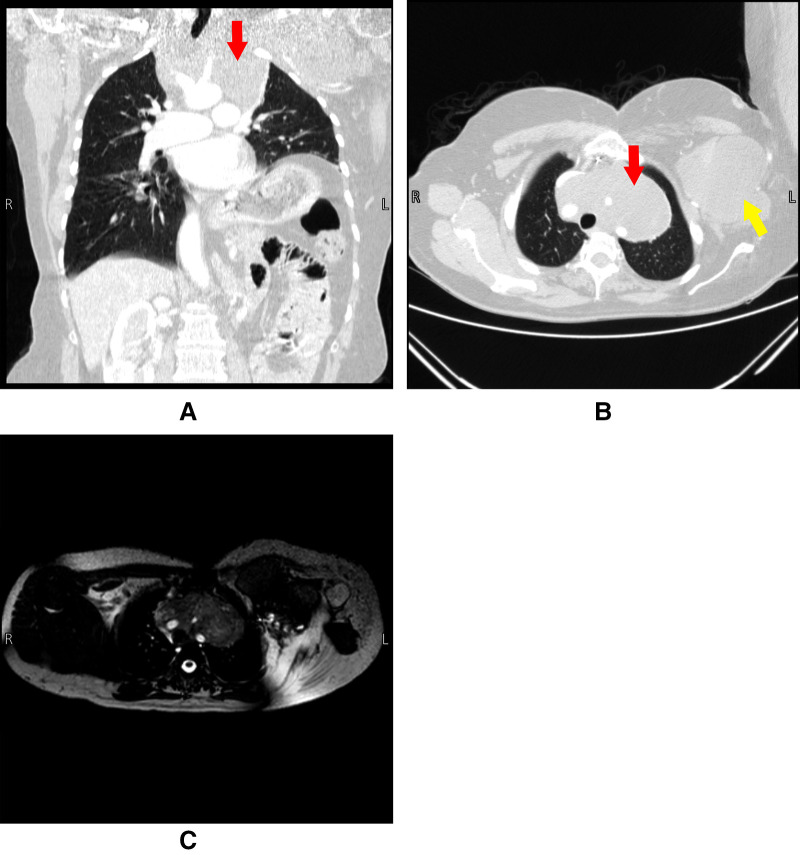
Panel (A) and (B) are CT scans done pre-operatively on 4/9/2021. Panel (C) is an MRI done pre-operatively on 8/9/2021. The figure shows a large anterior mediastinal mass engulfing the arch of the aorta and its branches. Red arrows indicate the mediastinal lesion, yellow arrow indicates the extrathoracic chest wall lesion.
